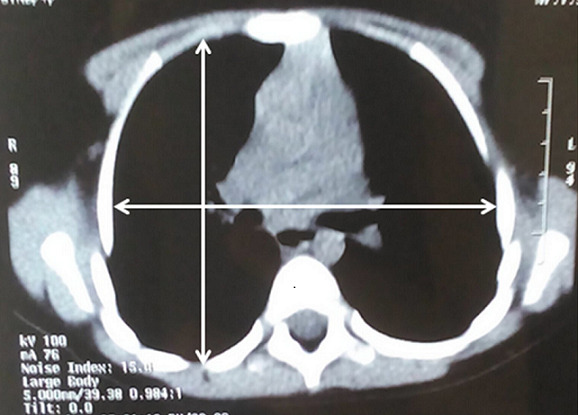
chest CT showing the overall decrease of transverse diameter of the rib cage
